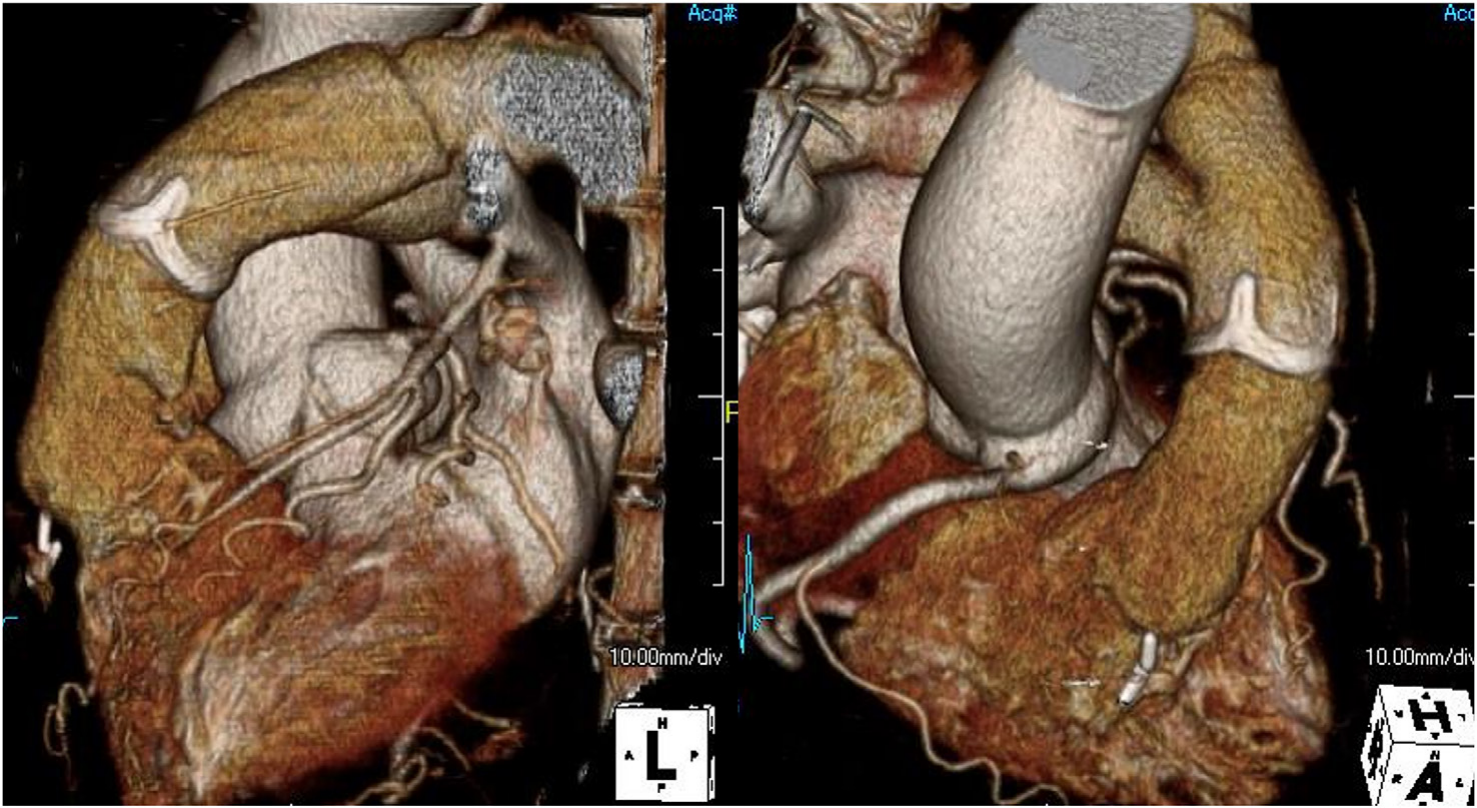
Postoperative contrast-enhanced computed tomography showed complete reconstruction of the RVOT and both pulmonary arteries using a prosthetic valve and vascular grafts. RVOT, right ventricular outflow tract
